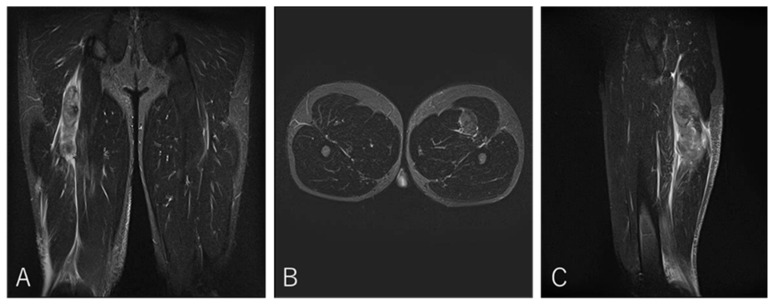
Case 5 MRI images: BFLH proximal MTJ injury, Type II. (A) (Coronal image): The tendon is extensively ruptured from the proximal portion of the MTJ with the CT of the BFLH. (B) (Axial image): prone position, Extensive haematoma is observed on the BFLH side of the CT. A similar appearance was observed from the proximal to the distal cross-sections. (C) (Sagittal image): Extensive haematoma was observed in the cross-section of the BFLH.
